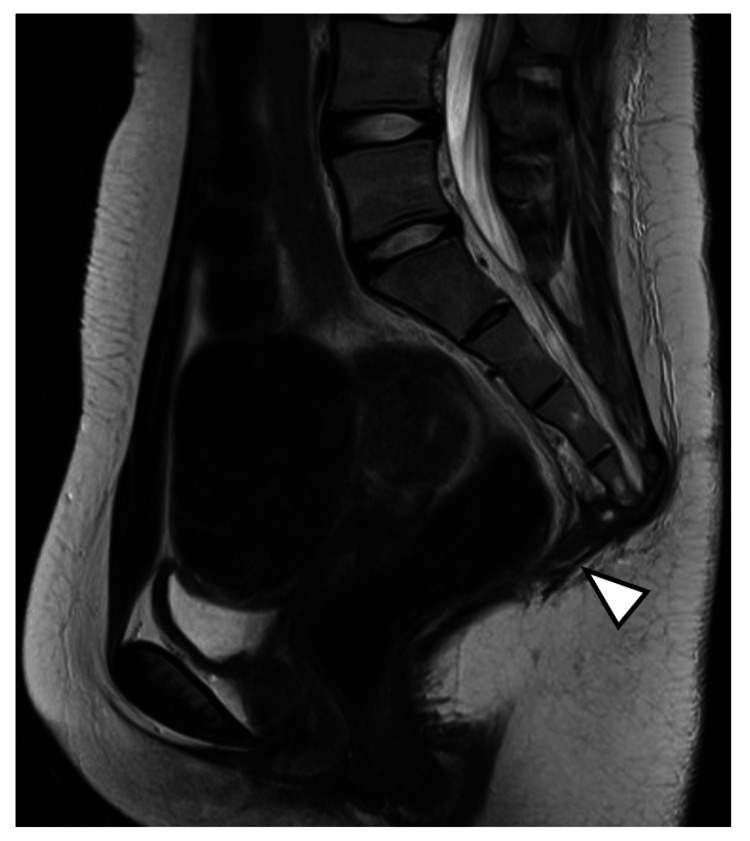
Post second surgery (MRI T2 sagittal). MRI one month postoperatively showed complete disappearance of the cyst (arrowhead).
